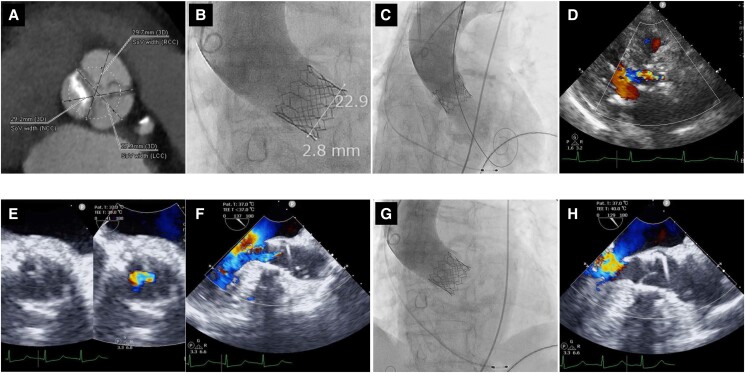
(*A*) Dense calcification at the non-coronary cups. (*B*) Final implantation position of valve. (*C*) Angiography and (*D*) transthoracic echocardiography demonstrating significant aortic insufficiency (AI). (*E*, *F*) Significant, central, transvalvular AI without prolapse by transoesophageal echocardiography (TEE). (*G*) Final angiography showing no AI. (*H*) Final TEE showing no AI.
